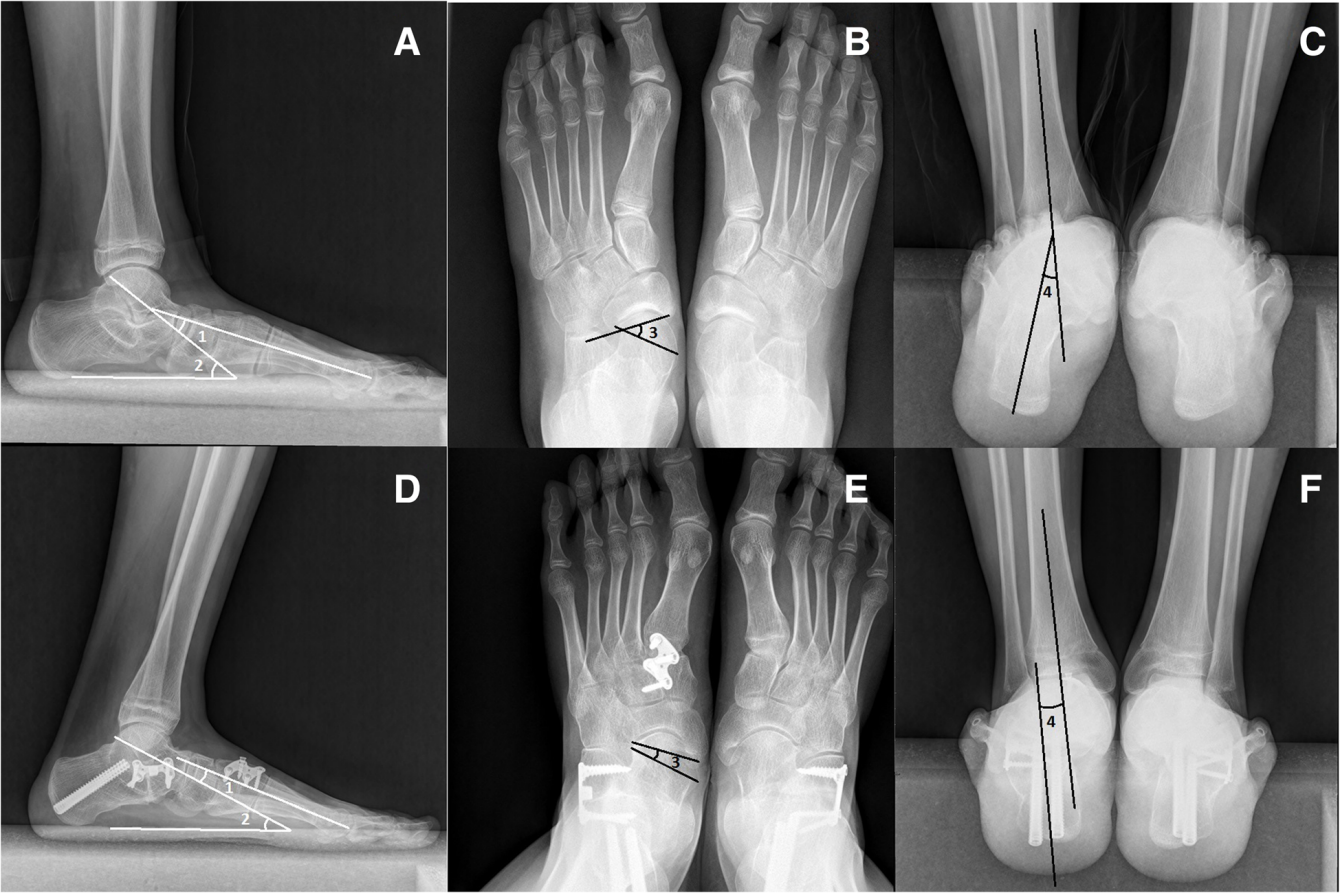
Correction of flexible flatfoot in a 14-year-old boy. Preoperative (a–c) and postoperative (d–f) weight-bearing radiographs show the realignment of flatfoot in lateral view, AP view, and hindfoot alignment view. 1, 2, 3, and 4 represent the talo-first metatarsal angle in lateral view, talar pitch angle, talonavicular coverage angle, and hindfoot valgus angle respectively
